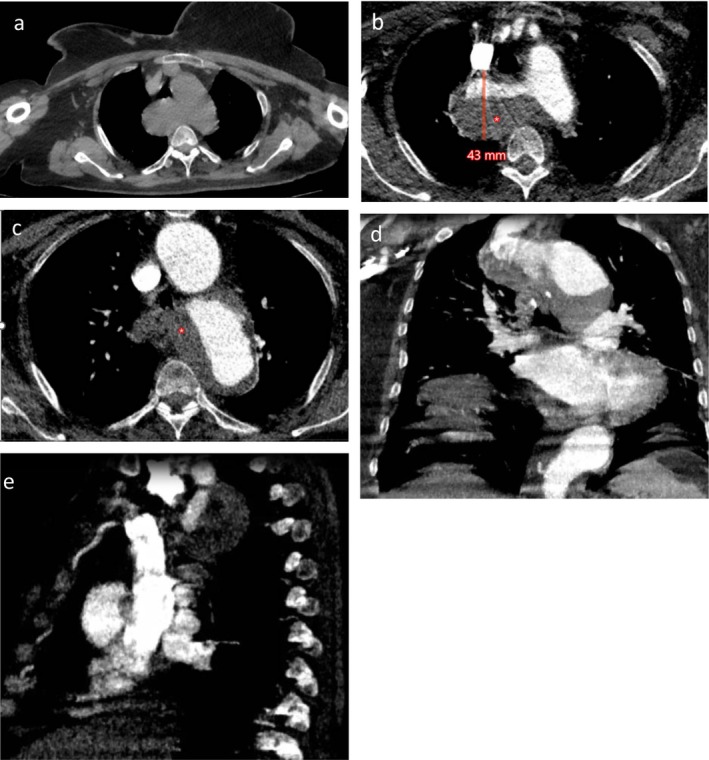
Thoracic aorta CTA at the patient's initial presentation. (a) Non‐contrast axial CT scan under soft tissue/mediastinal window settings, shows an aneurysmal dilation of the origin of ARSA. (b, c) Axial images of CT angiography (arterial phase) using vascular/heart window settings, show Kommerell aneurysm, with a maximum diameter of 43 mm, contains mural thrombus (*) and a dilated aortic arch. (d) Coronal reconstruction of arterial phase image under vascular/heart window settings, reveals the mural thrombus (*) in both the Kommerell aneurysm and the aortic arch. (e) Coronal reconstruction of arterial phase images using vascular/heart window settings shows a cross‐sectional view of ARSA and Kommerell aneurysm.
